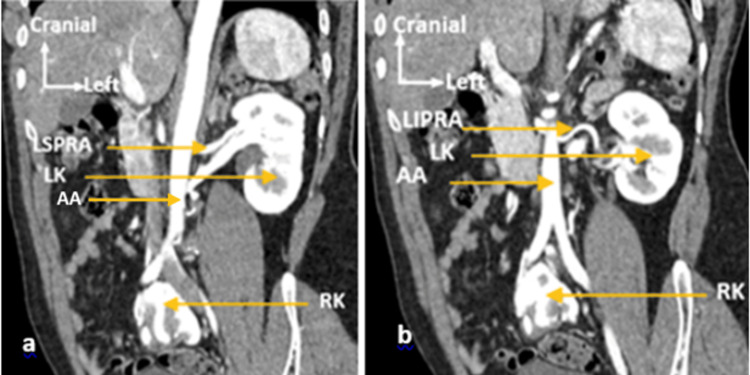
Frontal 2D CT section showing (a) the left superior polar renal artery (LSPRA) and (b) the left inferior polar renal artery (LIPRA). LK: Left kidney; AA: abdominal aorta; RK: right kidney
